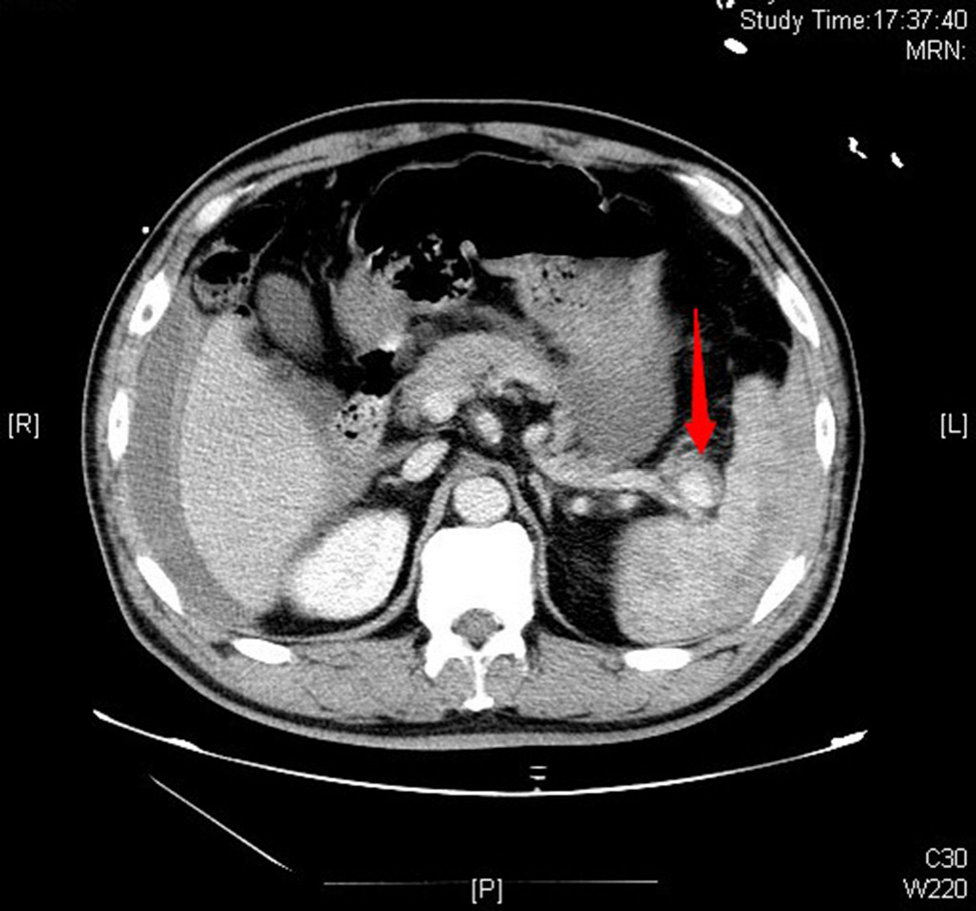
Contrast-enhanced CT of the abdomen showed contrast agent extravasation and ruptured splenic aneurysm (arrow).
